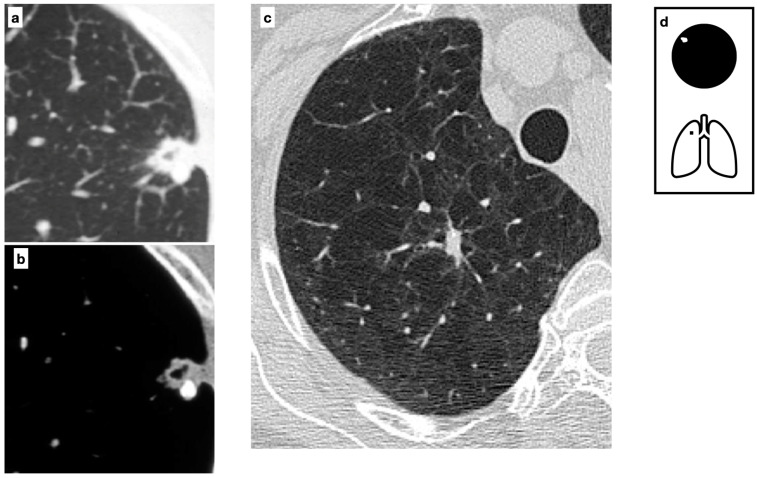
CT axial images demonstrate a peripheric lung nodule characterized by spiculated margins, small cavitation and an eccentric calcification; this lesion was a biopsy-proven adenocarcinoma (a,b). Heavy smoker patient with severe confluent centrilobular emphysema. HRCT shows a malignant nodule with a small eccentric calcification in the right upper lobe (c). A spiculated nodule with a relatively fast growth rate must always raise the concern for lung cancer in patients with smoking history. Pattern of calcification (d).
